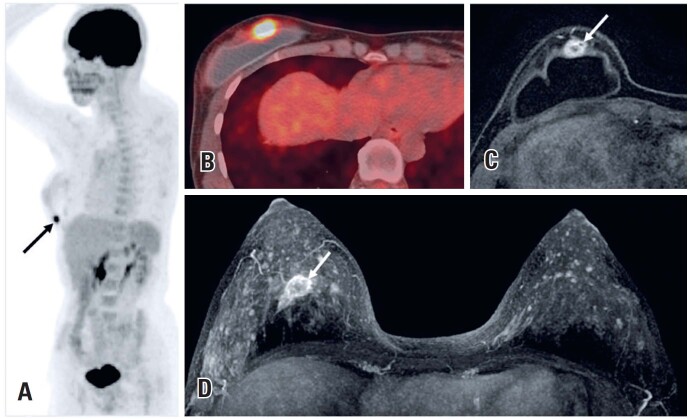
A 44-year-old woman under investigation for non-Hodgkin lymphoma underwent PET-CT (A and B). The PET-CT revealed increased uptake in the right breast (arrow). (C and D). Magnetic resonance imaging demonstrated an irregular mass with rim enhancement classified as BI-RADS 4. Breast biopsy confirmed invasive ductal carcinoma
